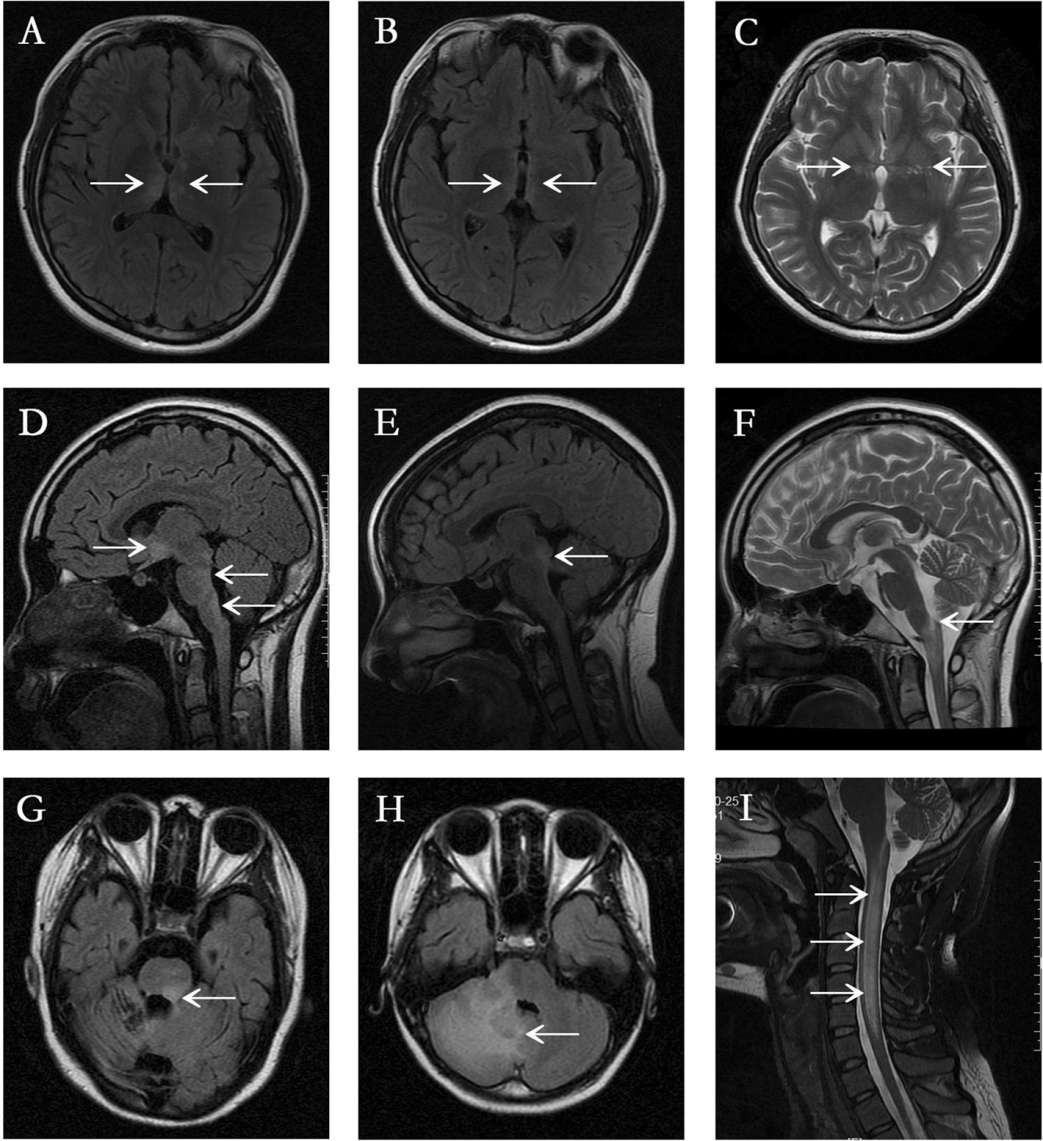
Representative magnetic resonance imaging (MRI) abnormalities (arrows) in NMOSD patients with autoimmune diseases. (A) Bilateral thalamic lesions; (B) Bilateral hypothalamus lesions; (C) Basal ganglia lesion; (D) Hypothalamus and periventricular lesions; (E) Aqueduct of the midbrain lesion; (F) Cervico-medullary lesion; (G) Pons lesion; (H) Cerebellum lesion; (I) Longitudinally extensive transverse myelitis (LETM) lesions.
